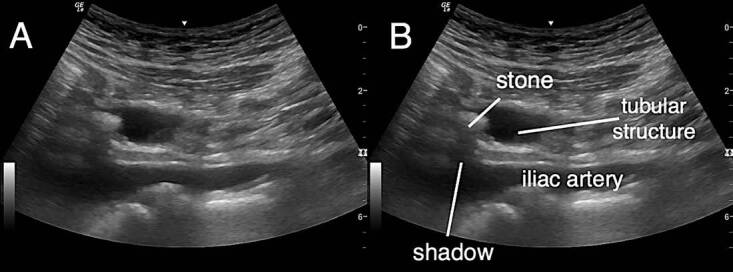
Ultrasound right lower quadrant, long axis, curvilinear probe A hyperechoic circular focus with acoustic shadowing is noted within a hypoechoic, non-peristalsing, non-compressible, tubular structure superior to the iliac vessels. A ureteral stone is identified as a hyperechoic structure, which demonstrates acoustic shadowing within the dilated ureter.
